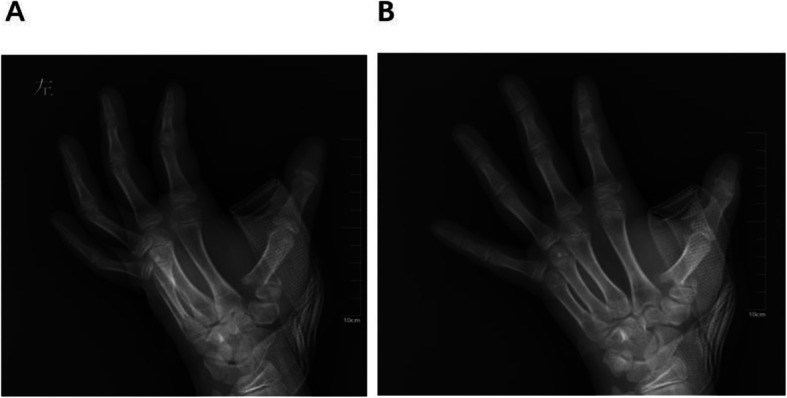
Typical thumb metacarpal base RW-C fracture of left hand. a AP X-Ray of left thumb preoperative. b lateral X-Ray of left thumb preoperative
